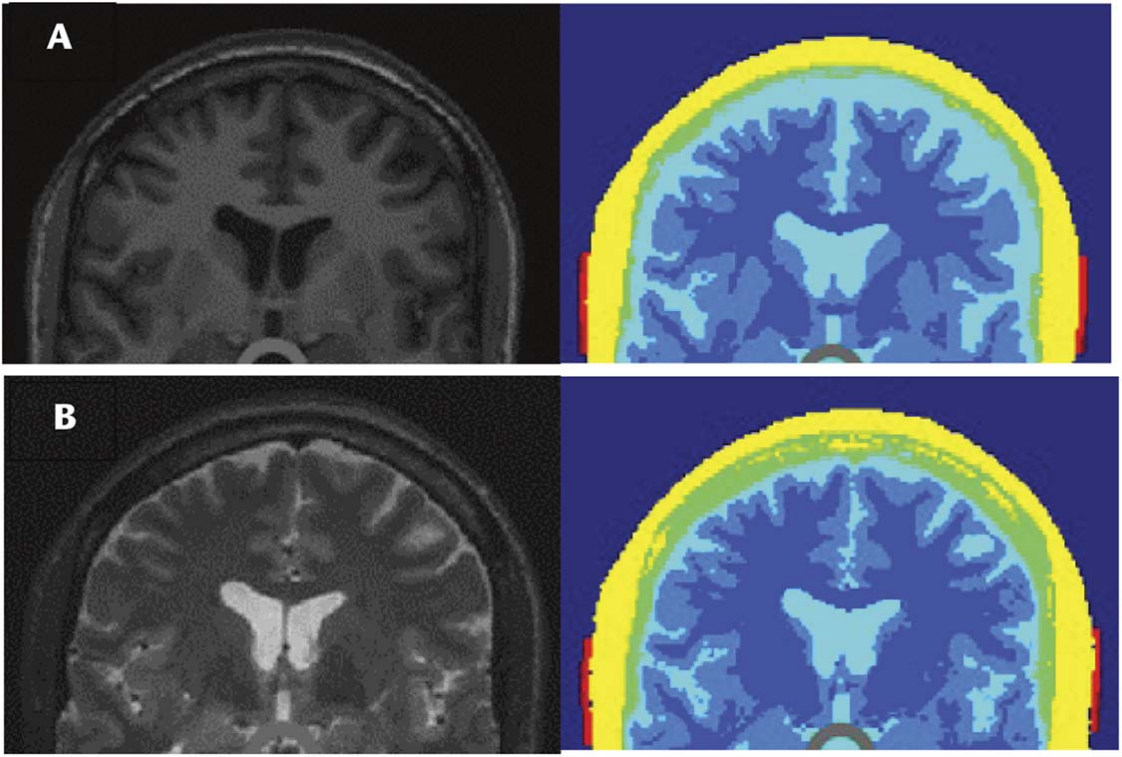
Coronal slice views of the (A) T1 MRI and (B) T1 and T2 MRI images and segmentation. The BT montage electrodes can be seen in red. The scalp (yellow), skull (green), CSF (cyan), gray matter (light blue), and white matter (dark blue) are demonstrated.
